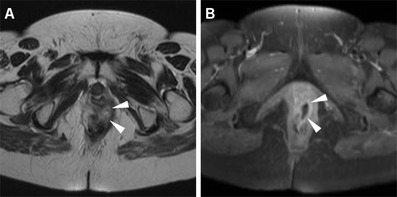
A 32-year-old HIV-positive woman with clinical diagnosis of anovaginal fistula. Axial T2-weighted (a) and post-contrast fat-suppressed axial T1-weighted (b) images show inhomogeneous anal tissue invading the left aspect of the vagina (*arrowheads*), with internal non-enhancing necrosis and peripheral enhancement. Biopsy diagnosed SCAC with superimposed infection
